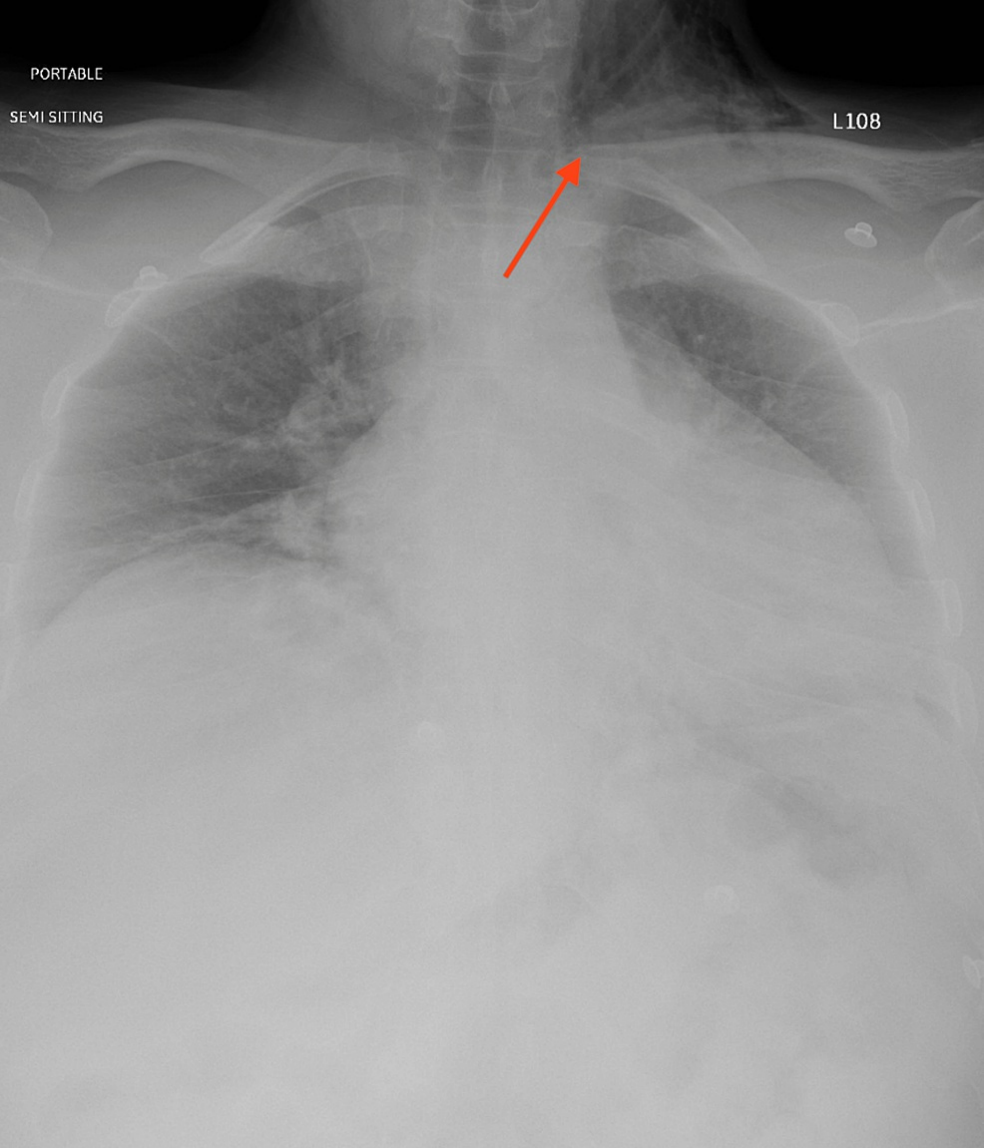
The plain anterior-posterior (AP) X-ray The arrow pointing to soft tissue lucency on the left side of the neck.
